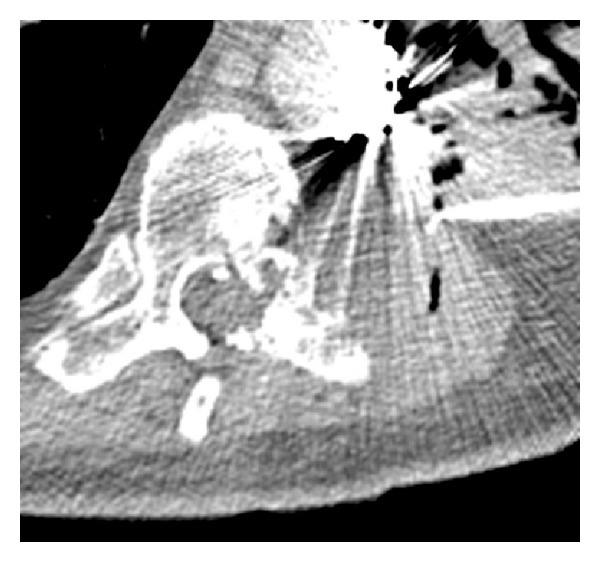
CT T-spine without contrast five months after irinotecan TACE and after pseudoaneurysm coil embolization. Note the metastatic lesion in the left paravertebral/chest wall area which has decreased in size, now measuring 5.5 cm × 5.2 cm.
